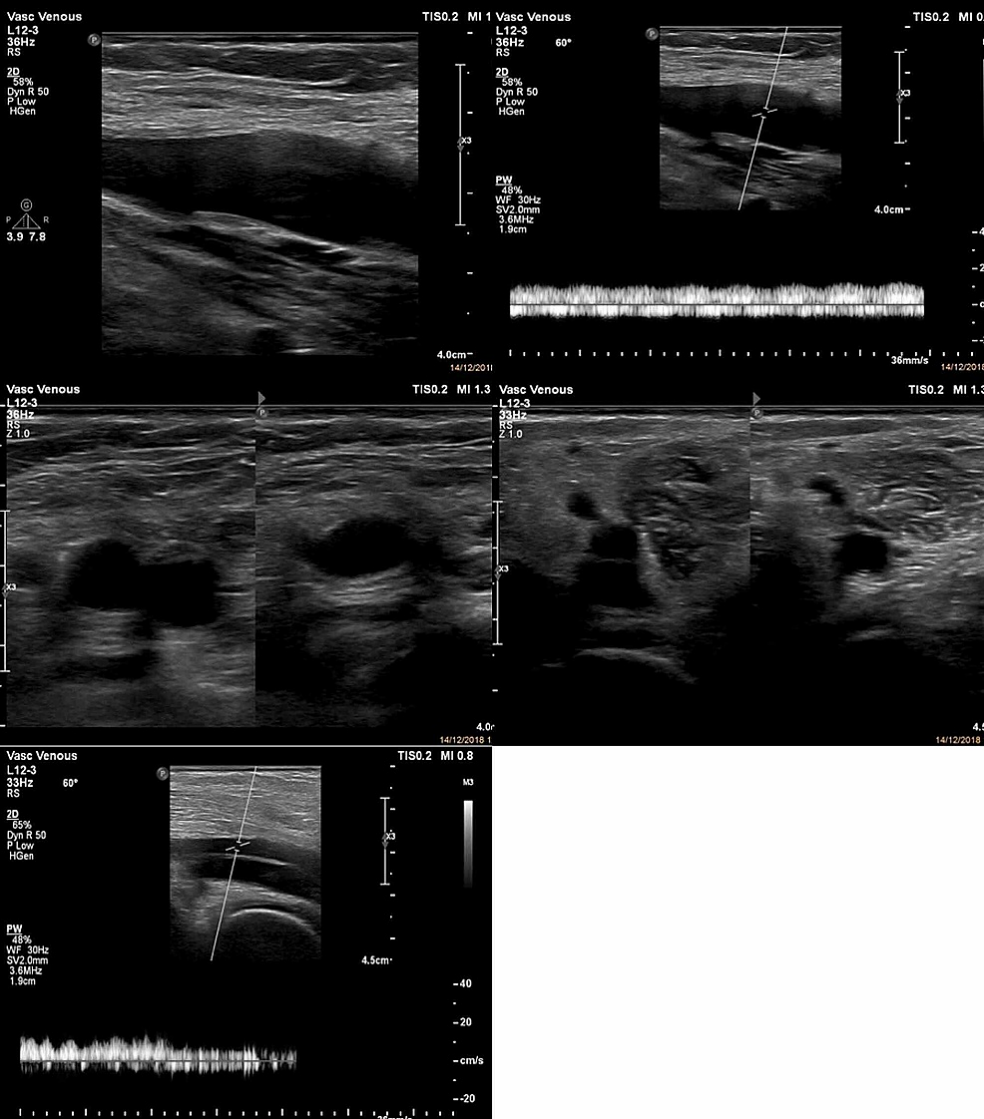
Follow-up duplex ultrasound of left leg after taking anticoagulant for more than one month showed normal flow of vein systems, no thrombus seen, and fully compressible of common femoral vein and popliteal vein.
